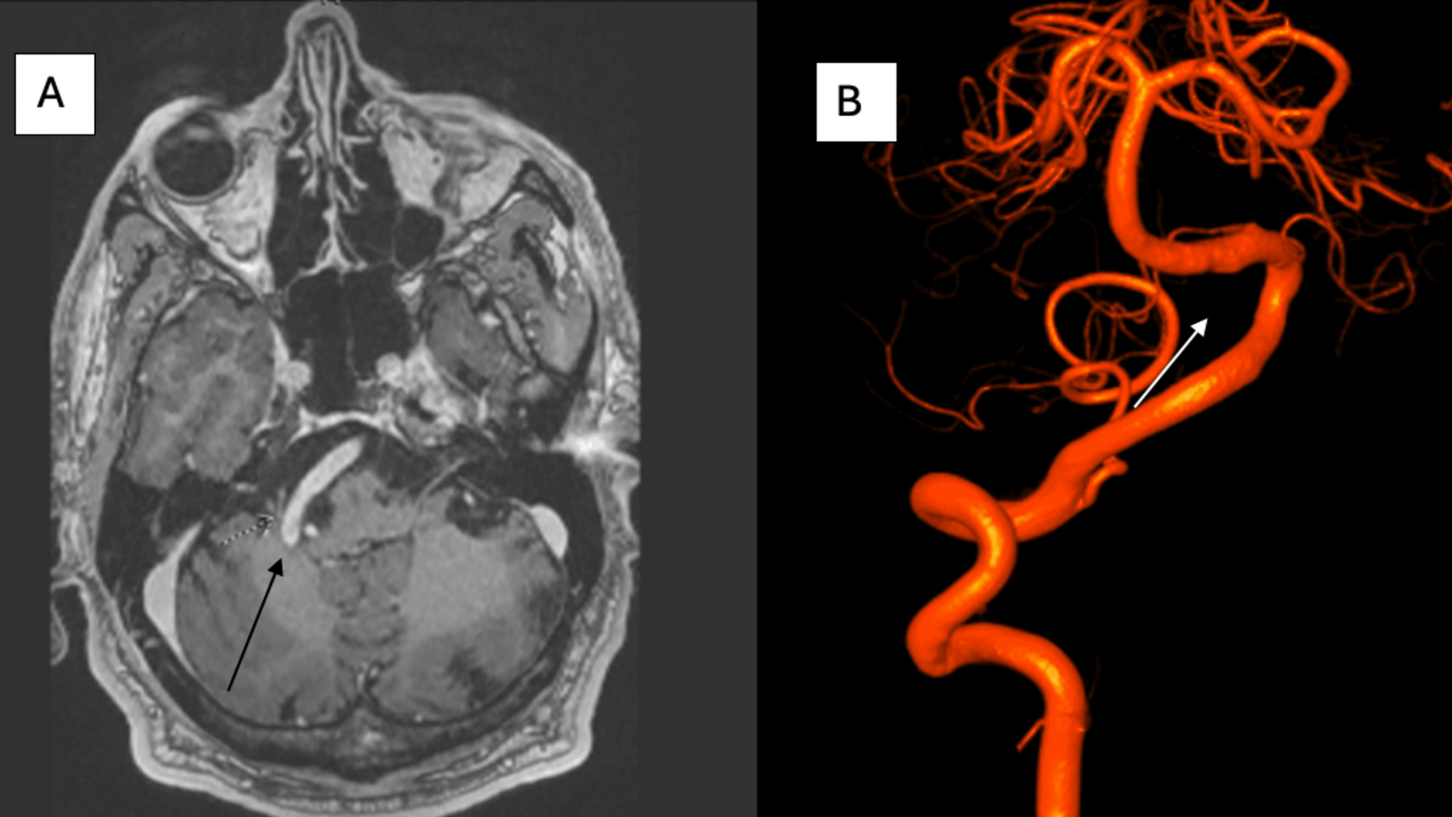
A. MRI of the brain with contrast showed dolichoectasia of the right vertebral artery, which crosses the midline to the left cerebellopontine angle and appears to exert a mass effect on the root entry zones of the left facial and vestibulocochlear nerves. B: Digital subtraction angiogram 3D construction showing tortuous elongated right vertebral with mild irregularities in lumen.
